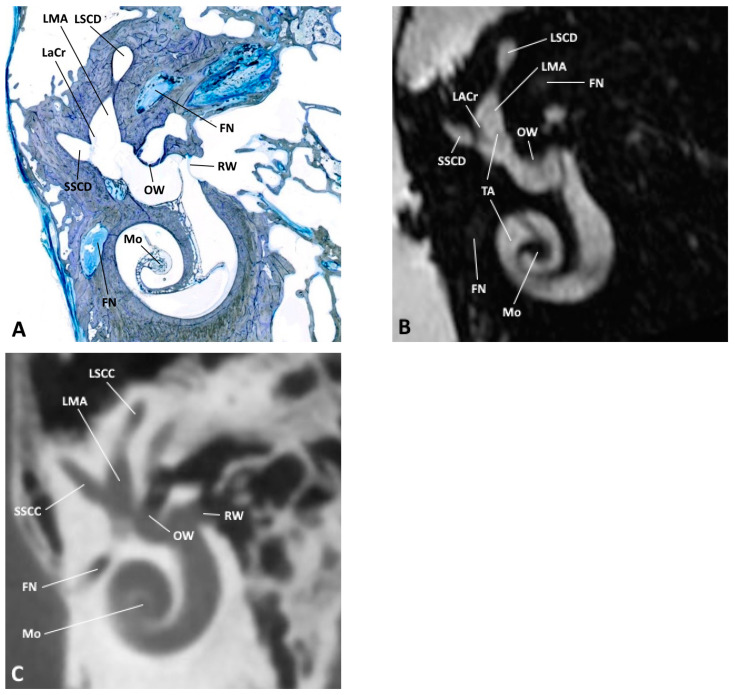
(A) Oblique anatomical section with the corresponding (B) MRI and (C) CBCT images; FN, facial nerve; LACr, lateral ampullary crest; LMA, lateral membranous ampulla; LSCC, lateral semicircular canal; LSCD, lateral semicircular duct; Mo, modiolus; OW, oval window; RW, round window; SSCC, superior semicircular canal; SSCD, superior semicircular duct; TA, truncation artifact.
